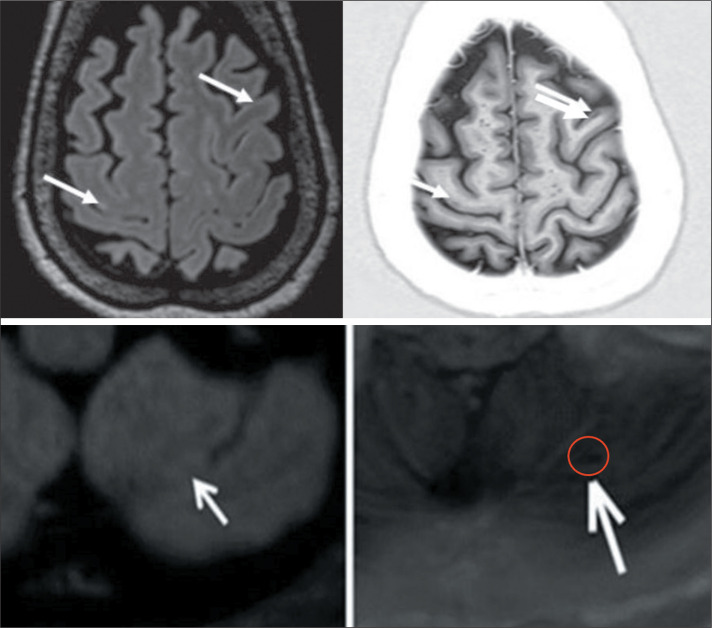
Lesions detected only on PSIR sequences. Axial 3D FLAIR (left) and axial
